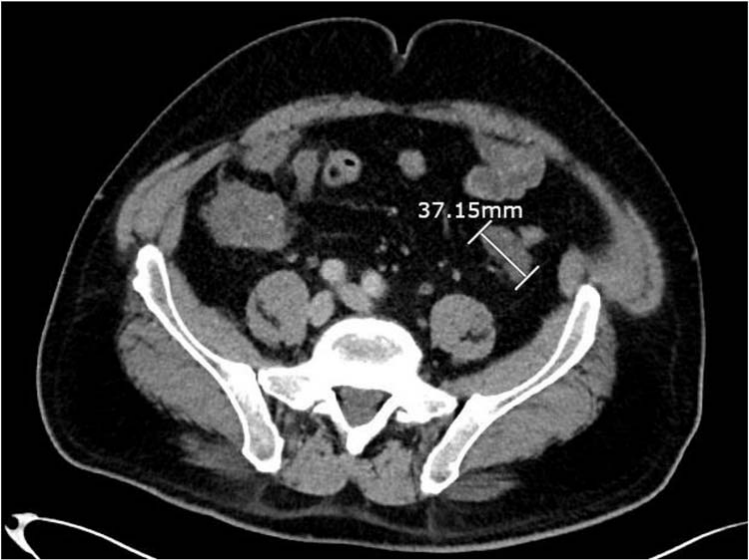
Axial CT abdomen with contrast demonstrating a peritoneal deposit posterior to the descending colon in the left iliac fossa, measuring 37.15 mm.
